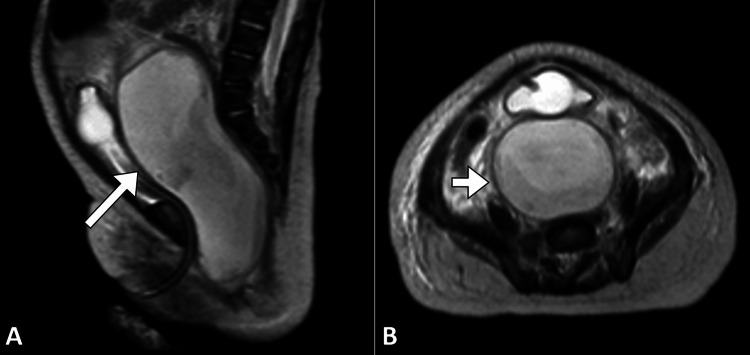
MRI abdominopelvic sagittal (A) and axial (B) T2-weighted images showing a distended uterus and vagina filled with fluid (arrow), consistent with an obstructed hemivagina.
